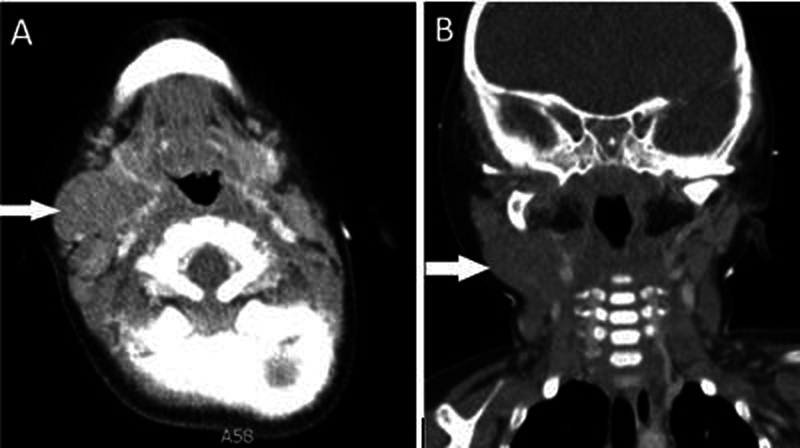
Intravenous contrast-enhanced CT neck (
